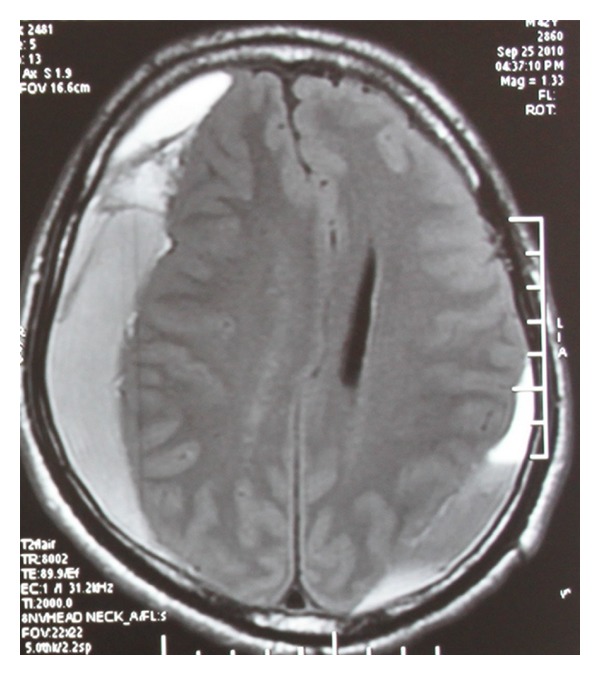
Post-ECT cranial noncontrast magnetic resonance imaging showing bilateral asymmetric extensive acute subdural hematoma extending over right frontoparietal and left parietal areas with mass effect and midline shift to left.
